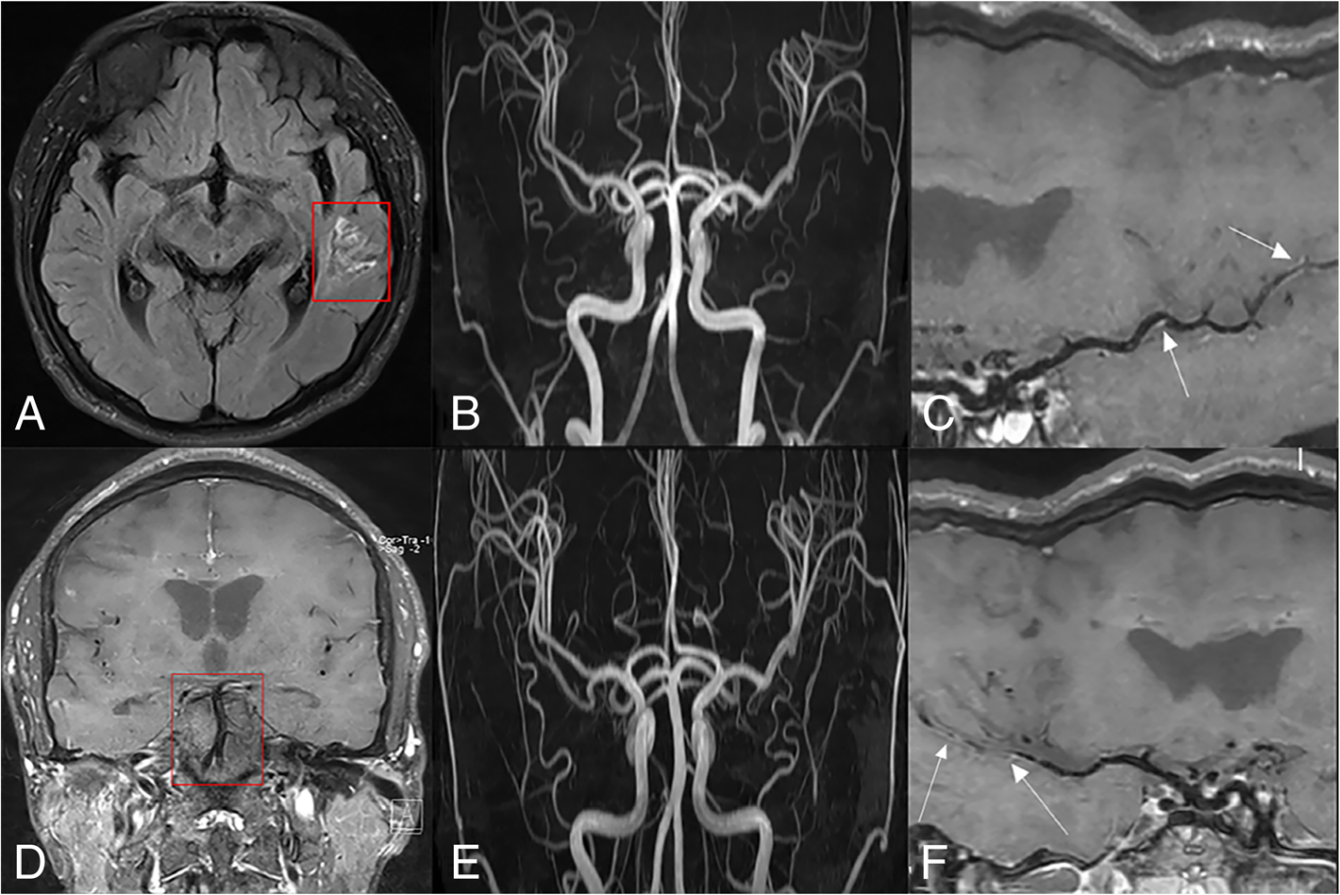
a: MR-FLAIR image of the brain, two months later after initial treatment, with new abnormal signals in the left temporal lobe. b: No obvious abnormality in 3D-TOF-MRA. c: The black-blood sequence image with an abnormal enhancement of the left middle cerebral artery (arrow mark). d: The black-blood sequence image detecting that abnormal enhancement of the basilar artery and bilateral posterior cerebral artery had disappeared. e: No obvious abnormality in 3D-TOF-MRA four months after initial treatment. f: The black-blood sequence image with local thickness and abnormal enhancement of the right middle cerebral artery four months after the initial treatment (arrow mark)
